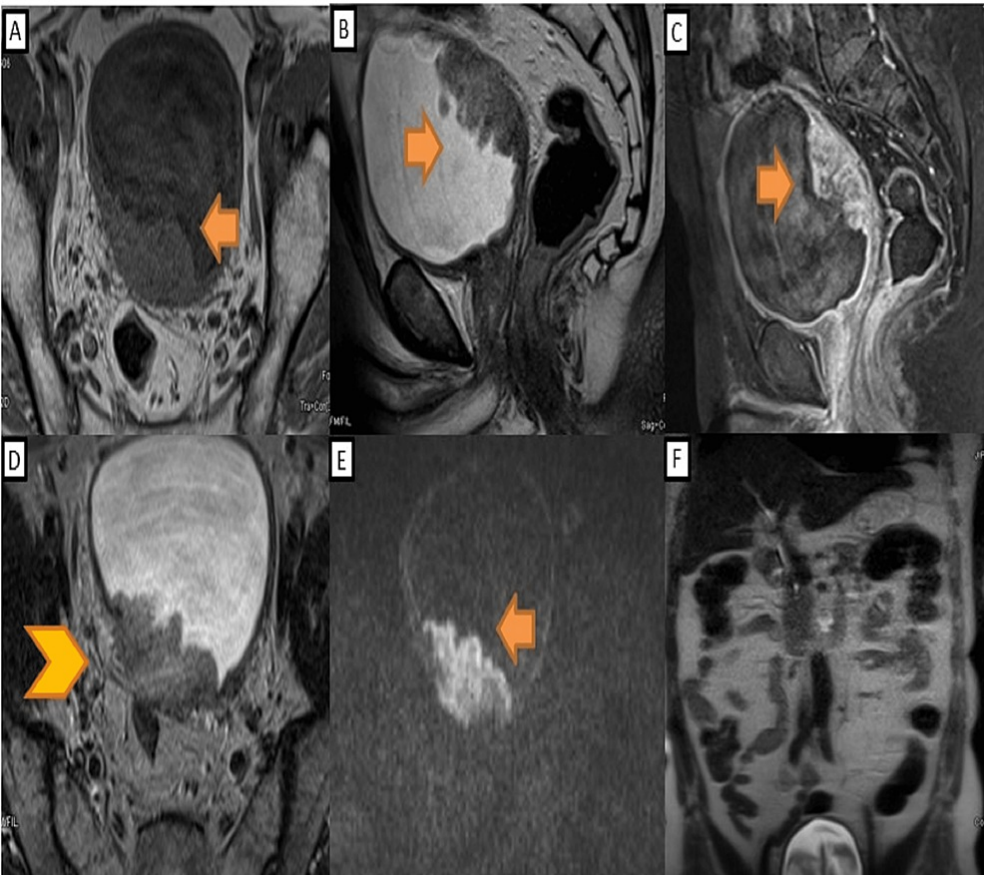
MRI (Magnetic Resonance Imaging) of the lesion A) T1 axial section shows an ill-defined irregular isointense mass lesion of 5x3.5x2.5 cm on the right lateral and posterior wall of the bladder (arrow); B) T2 sagittal section shows hypo to the intermediate signal intensity of the mass lesion (arrow); C) T1 post-contrast fat-suppressed image shows heterogeneous enhancement of the lesion (arrow); D) T2 axial section shows peri-vesical fat invasion and loss of fat plane with the anterior wall of the rectum (arrowhead); E) Diffusion Weighted image shows the urinary bladder mass (arrow); F) T2 HASTE (Half Fourier Single-shot Turbo spin-Echo) shows no lymph node or solid organ metastasis. The lesion shows VIRADS 5 (Vesical Imaging-Reporting and Data System).
